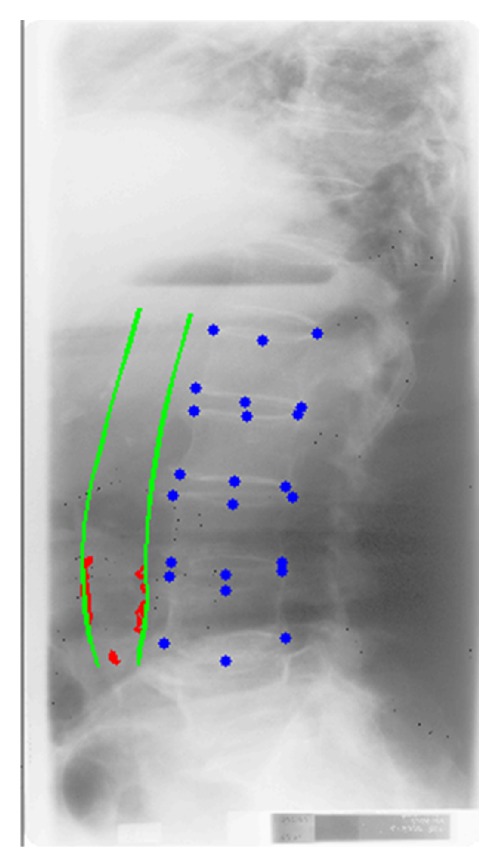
A manual annotation of an X-ray: in blue we see distinct vertebra points, in green the aorta wall, and in red the calcifications.
